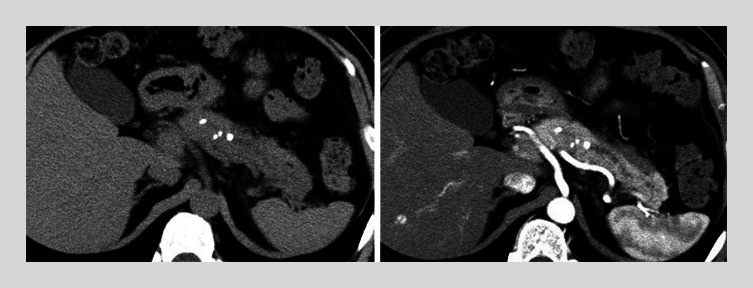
Abdominal computed tomography (CT) demonstrates pancreatic stones in the pancreatic body (left). The arterial phase of contrast-enhanced CT demonstrates pancreatic stones with dilation of the main pancreatic duct and inflammation around the pancreas (right).
